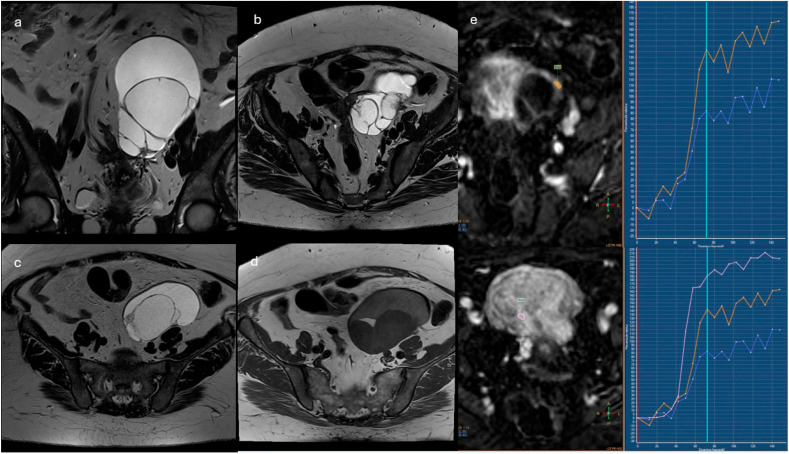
Left adnexal multiloculated cystic mass (a–d), with different signal intensity of the fluid in the loculi, especially in T1w sequences. In DCE-perfusion ROI were placed in thickened septa and wall ((e), orange and blue curves) and in the myometrium ((e), pink curve). The lesion was classified as O-RADS MRI Score 4 and resulted in mucinous cystoadenofibroma.
